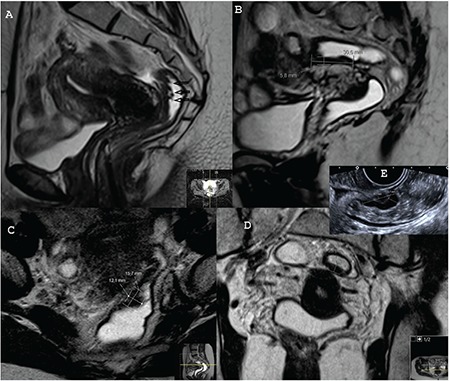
A) Sagittal T2W image of patient 3 in the previous magnetic resonance imaging (MRI) scan. There seems to be wall thickening with an Indian headdress sign (arrows). However, after the administration of the rectal fluid and the anti-peristaltic agent, the actual lesion is found in another segment of the sigmoid bowel, B) Sagittal T2W image of patient 3 in the present MRI scan. The actual place of the sigmoid bowel involvement was in a different segment in contrast to the previous MRI scan (Figure 5A), C) Axial T2W image of patient 3 in the present MRI scan. The lesion is measured, D) Coronal T2W image of patient 3 in the present MRI scan. The lesion is measured, E) Transvaginal ultrasound in patient 3. The endometriotic intestinal nodule is measured. There is regular thickening in the muscular propria
